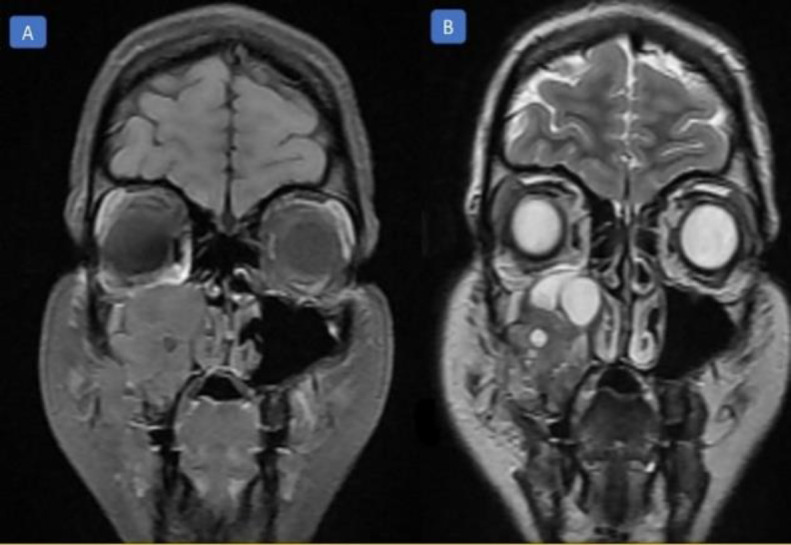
Coronal T1 and T2 weighted magnetic resonance imaging: A) Low-signal soft tissue mass in the right maxillary sinus, B) A non-homogenous lesion with fluid-fluid levels in the right maxillary sinus
